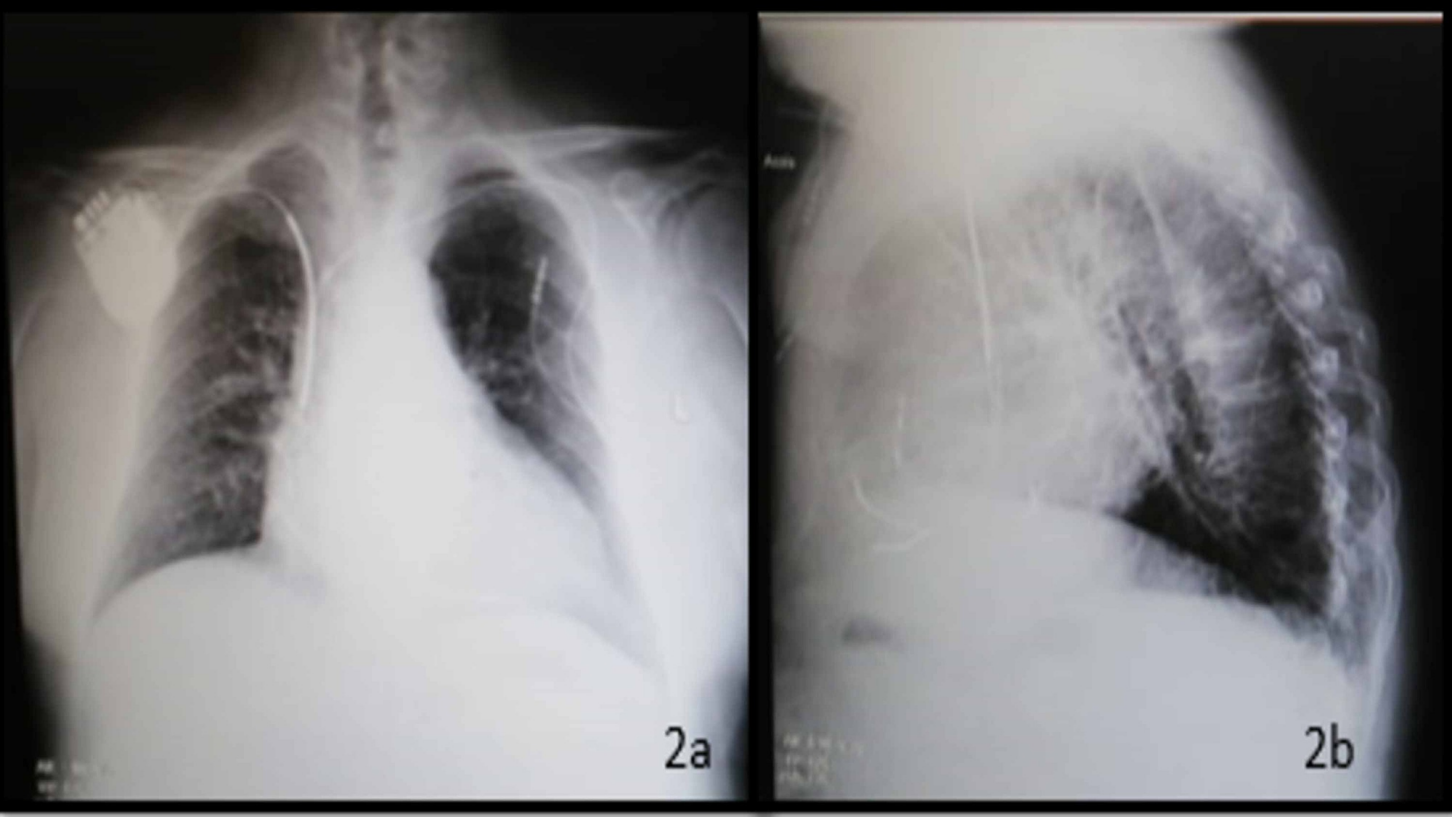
Device implantation on the right side 2a: chest X-ray posteroanterior (PA) view 2b: chest X-ray lateral view
